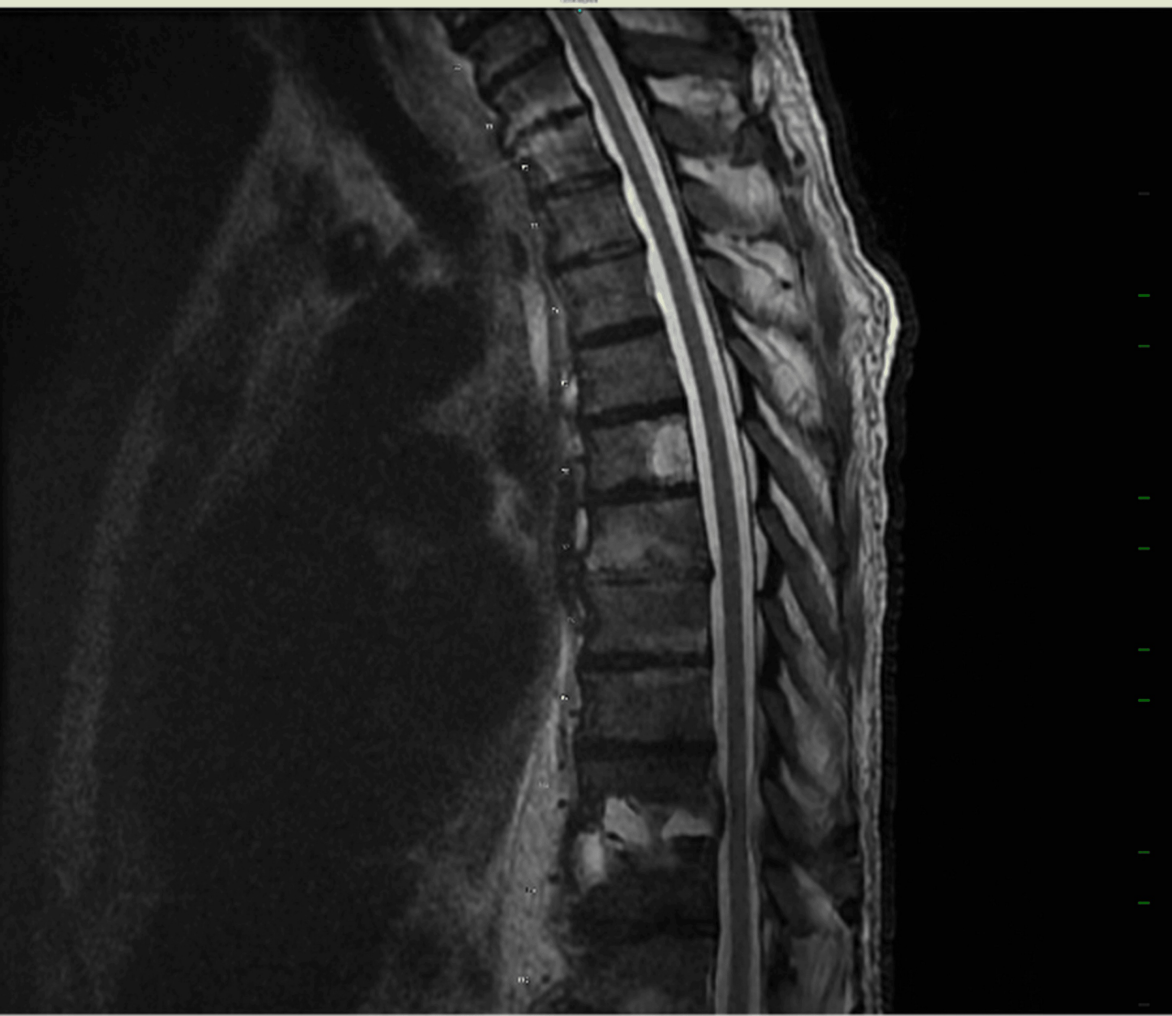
MRI of the thoracic spine without contrast Advanced discitis and osteomyelitis pattern at the T10-T11 level noted. There is suggestion of slight increase in epidural enhancement at the T10-T11 level with slight increased effacement of the thecal sac at this level. A gadolinium enhancement is visible on T6 displaying a large hemangioma that is not clinically significant.
